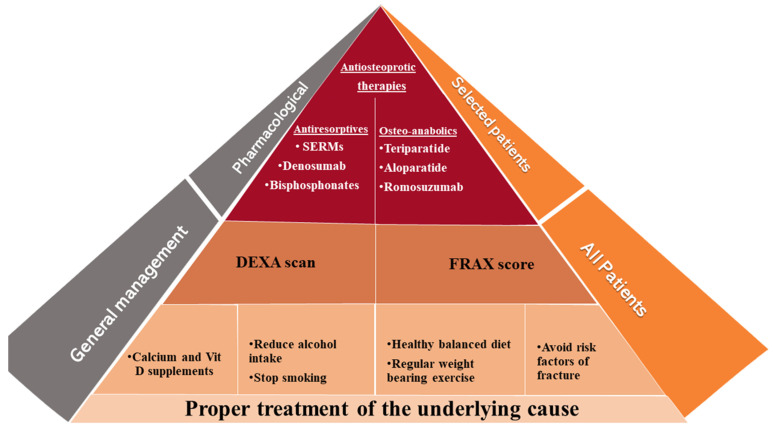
Approach for prevention and management of secondary osteoporosis. Correction of the underlying causes of secondary osteoporosis is the cornerstone of prevention and treatment. All patients can benefit from non-pharmacological intervention, DEXA scan and assessment of fracture risk. Anti-osteoporotic medications (antiresorptives and osteoanabolics) can be used in selected cases with high fracture risk. DEXA: dual-energy X-ray absorptiometry, FRAX: fracture-risk algorithm, SERM: Selective estrogen receptor modulators.
